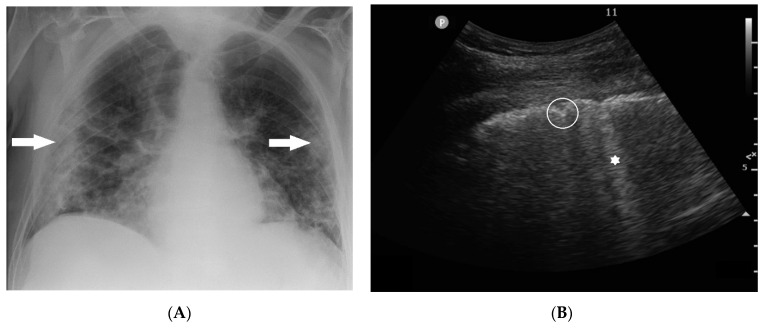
(A) Chest X-ray and (B) lung ultrasound of an 81-year-old patient with consolidations at the periphery of both lungs (arrow) in CXR and corresponding loss of aeration with well-defined B-lines (asterisk) and pleural line irregularities (circle) in LUS at presentation in hospital. LUS was performed with a convex C6-2 probe with a 2–6 MHz frequency range.
